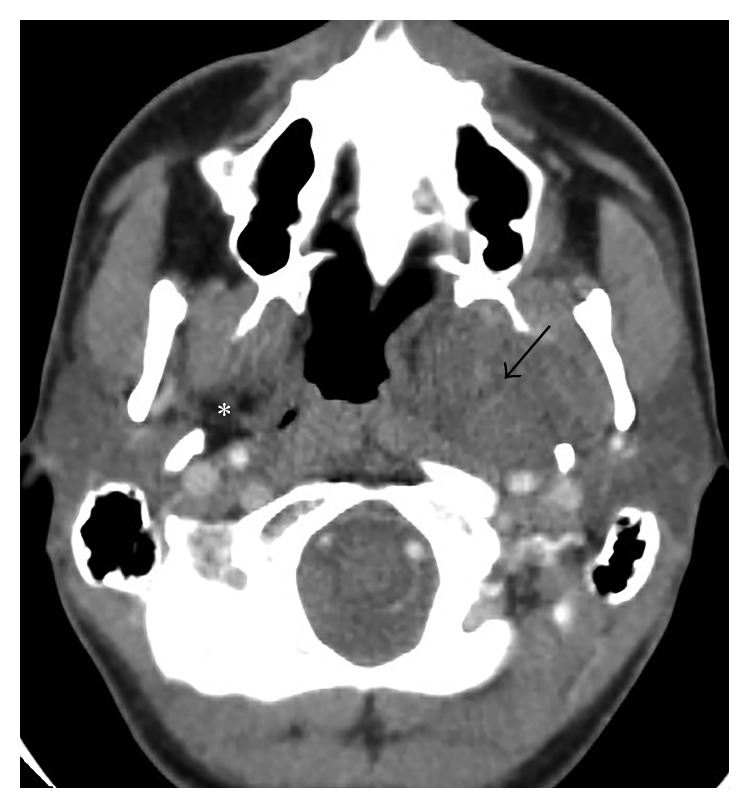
The contrast-enhanced axial CT scan shows the nonhomogeneous tumor within the left parapharyngeal space with a narrowing pharyngeal lumen (arrow). Note the normal fat contour in the right prestyloid compartment (asterisk).
